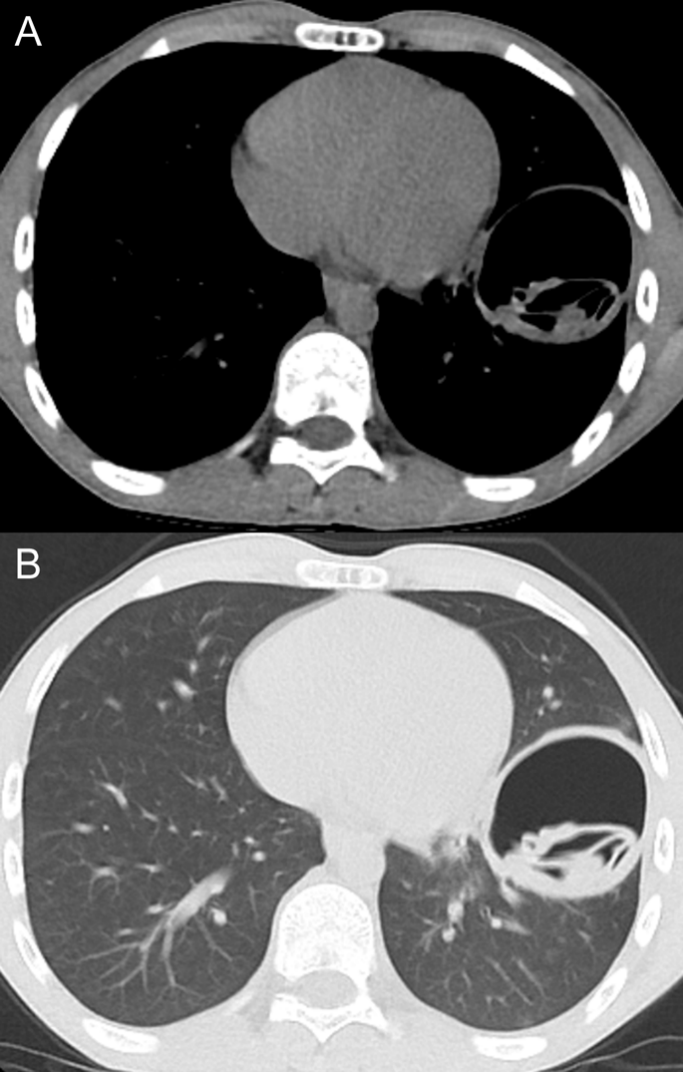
In a 14-year-old male, images of the air and laminar membrane in the cavity of the ruptured cyst in the left lower lobe are seen on the thorax computed tomography mediastinum (a) and parenchyma sections (b).
